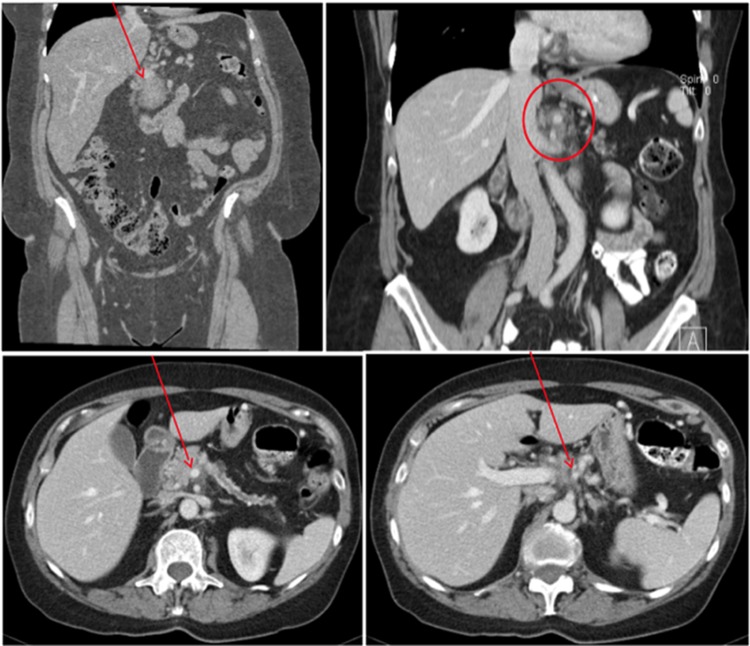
Initial CT scan at presentation showing a locally advanced tumor (arrow) of the proximal pancreatic body (upper left) and dilatation of the main pancreatic duct with involvement of the major visceral vessels, including the celiac artery (upper right), portal vein (lower left; arrow), and splenic artery and vein (lower right; arrow).
